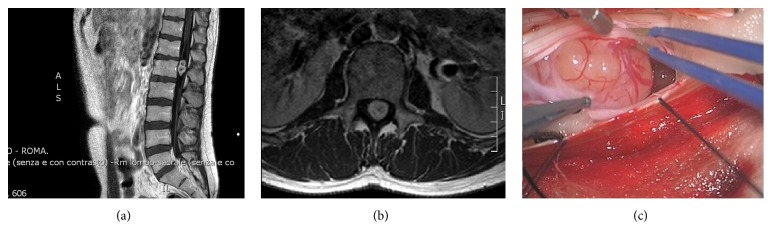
(a) Sagittal and (b) axial preoperative contrast enhanced MRI scan. (c) Intraoperative image of the lesion at gross examination resembles an ordinary schwannoma. Note the “target” pattern in (a) and the finely heterogenous contrast enhancement in (b), consistent with the typical aspect of an ancient schwannoma.
